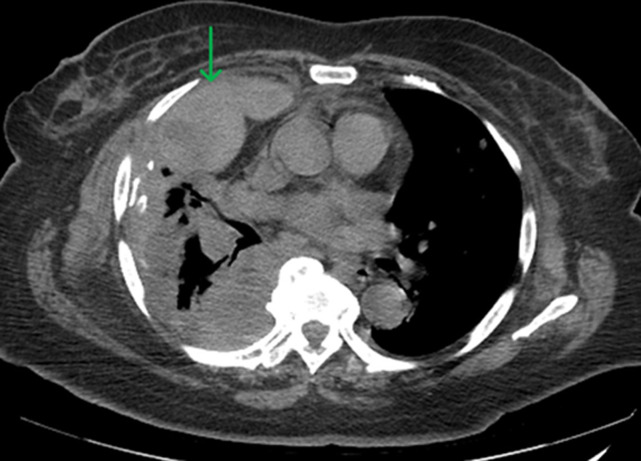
contrast-enhanced chest CT angiogram performed for acute respiratory exacerbation, revealing large multiloculated right pleural effusion with lobar atelectasis and right hemothorax volume loss, variable sized nodules in the left lung suspicious for metastasis and suspected right upper lobe “pneumonectomy” like changes with adjacent solid appearing 10 cm bilobed lesion concerning for a pulmonary malignancy (green arrow)
